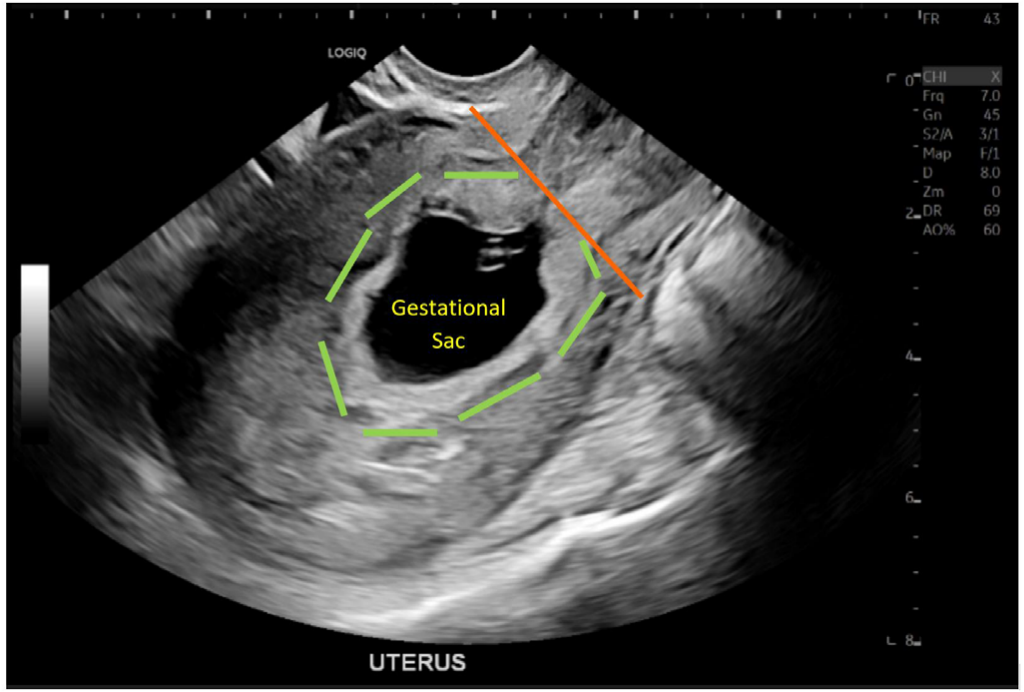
Outpatient obstetric ultrasound shows mid‐sagittal view of the uterus at 6 weeks 6 days of gestation (based on last menstrual period). Gestational sac lies in a low position within the uterus (green outline) with irregular contour above the level of the internal os (orange line). There is a yolk sac and a fetal pole. Crown‐rump length measures 8.3 mm, yolk sac 1.8 mm, and fetal heart rate was low at 74 bpm. This was originally reported as intra‐uterine pregnancy of uncertain viability with poor prognostic factors of fetal bradycardia, low‐position and irregular gestational sac outline.
